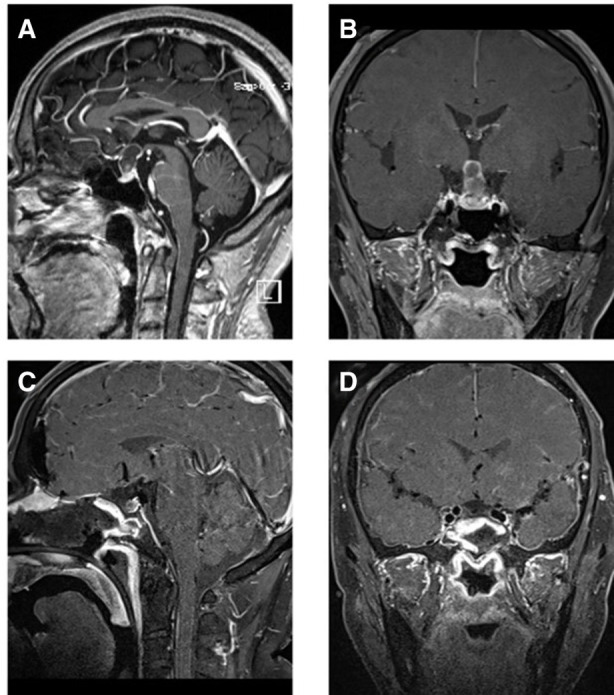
T1-weighted MRI image with contrast enhancement of a suprasellar craniopharyngioma. (A) Preoperative sagittal view. (B) Preoperative coronal view. (C) Postoperative sagittal view. (D) Postoperative coronal view. Note in (C) and (D) the autologous fat employed intradurally to fill the empty space within the suprasellar after tumor removal.
